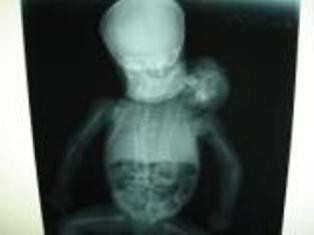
A plain X-ray of the twins showing two separate spines.
